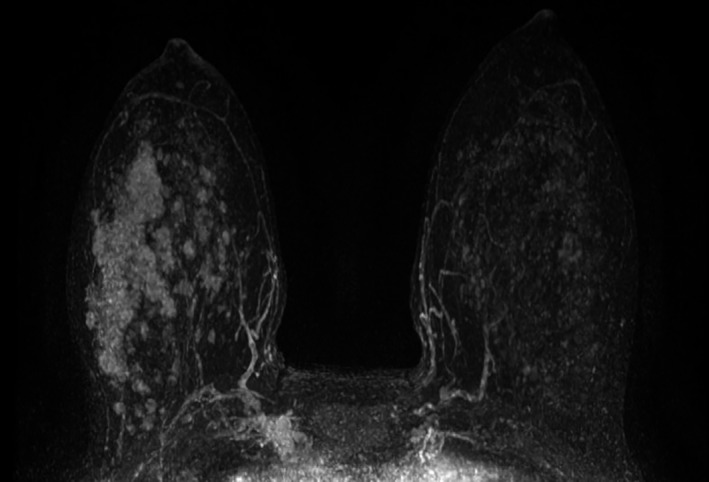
Maximal intensity projection from MRI with subtracted axial postgadolinium spoiled gradient‐echo images demonstrates a large mass in the lateral right breast, corresponding to the biopsy‐proven ILC, with multiple small masses extending medially
